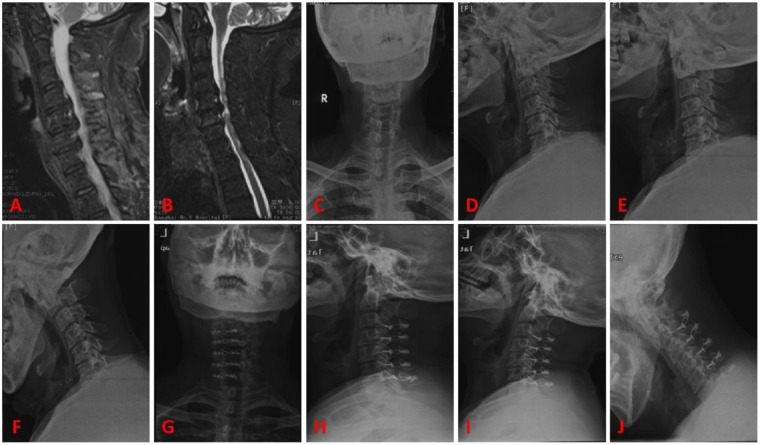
Typical case 1. (A,B) Preoperative sagittal MRI images, showing varying degrees of disc protrusion at the C4/5, C5/6, and C6/7 levels. (C,D) Preoperative anterior-posterior x-rays, showing loss of cervical lordosis. (E,F) Preoperative flexion-extension x-rays, showing instability of the C2 vertebra. (G,H) Postoperative follow-up lateral x-rays, showing good position of the internal fixation. (I,J) Postoperative follow-up flexion-extension x-rays, showing no significant changes in the instability of the C2 vertebra.
